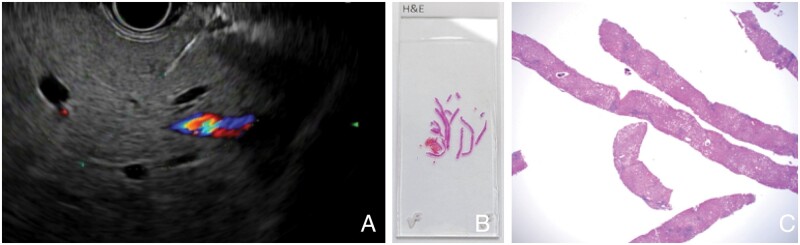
Endoscopic ultrasound (EUS)-guided liver biopsy. (A) A 19-gauge fine-needle biopsy (FNB) needle with transgastric access is used to access the liver. Careful attention is made to avoid any vasculature. Following the biopsy, the needle is slowly withdrawn and the tract is examined for any evidence of bleeding. (B) Gross image from a 19-gauge FNB with excellent core samples with intact portal tracts. (C) ×20 image of FNB core samples.
